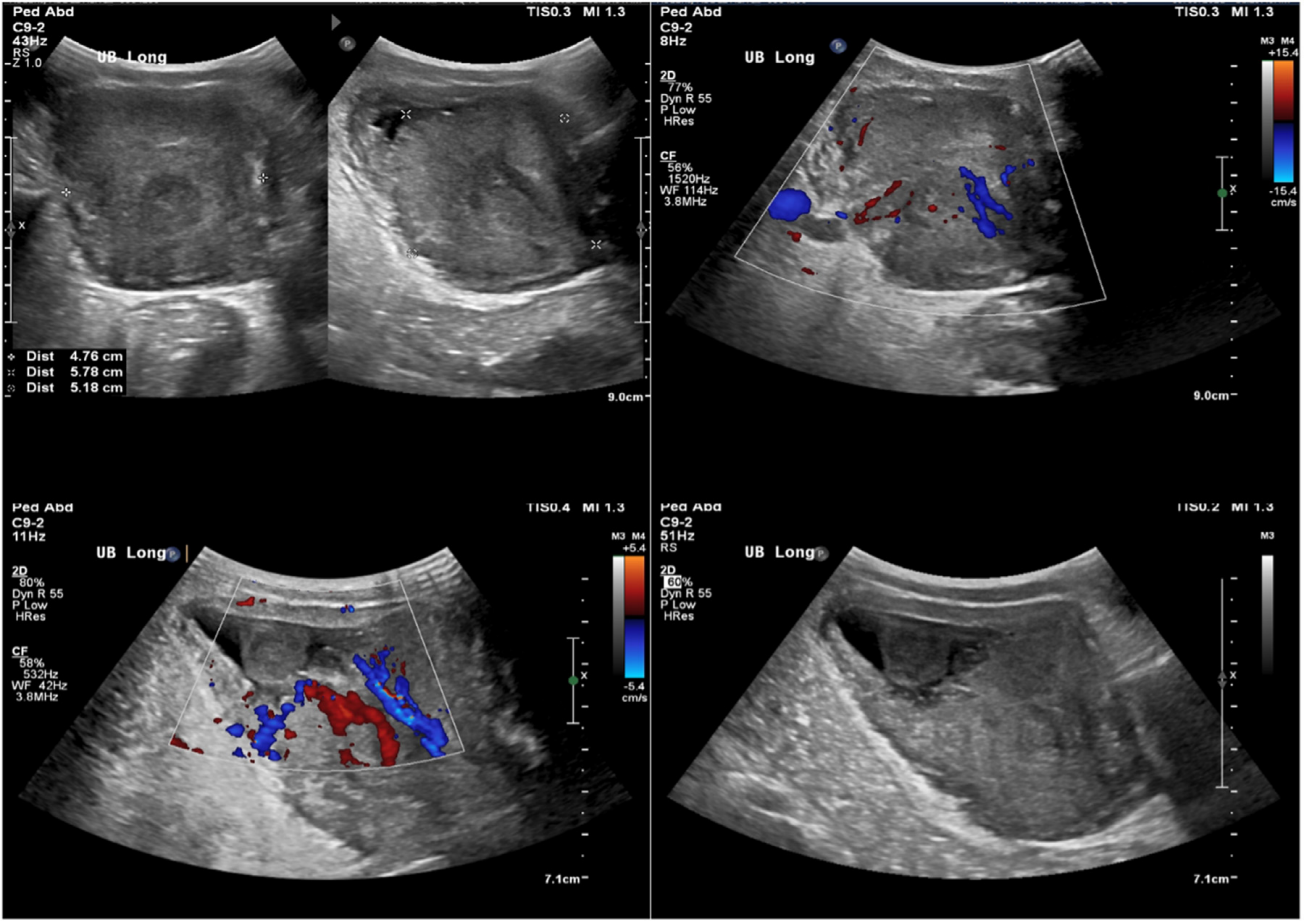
Pelvic ultrasound showed hypervascular large mass with difficulty to define its origin, either from bladder or prostate.
